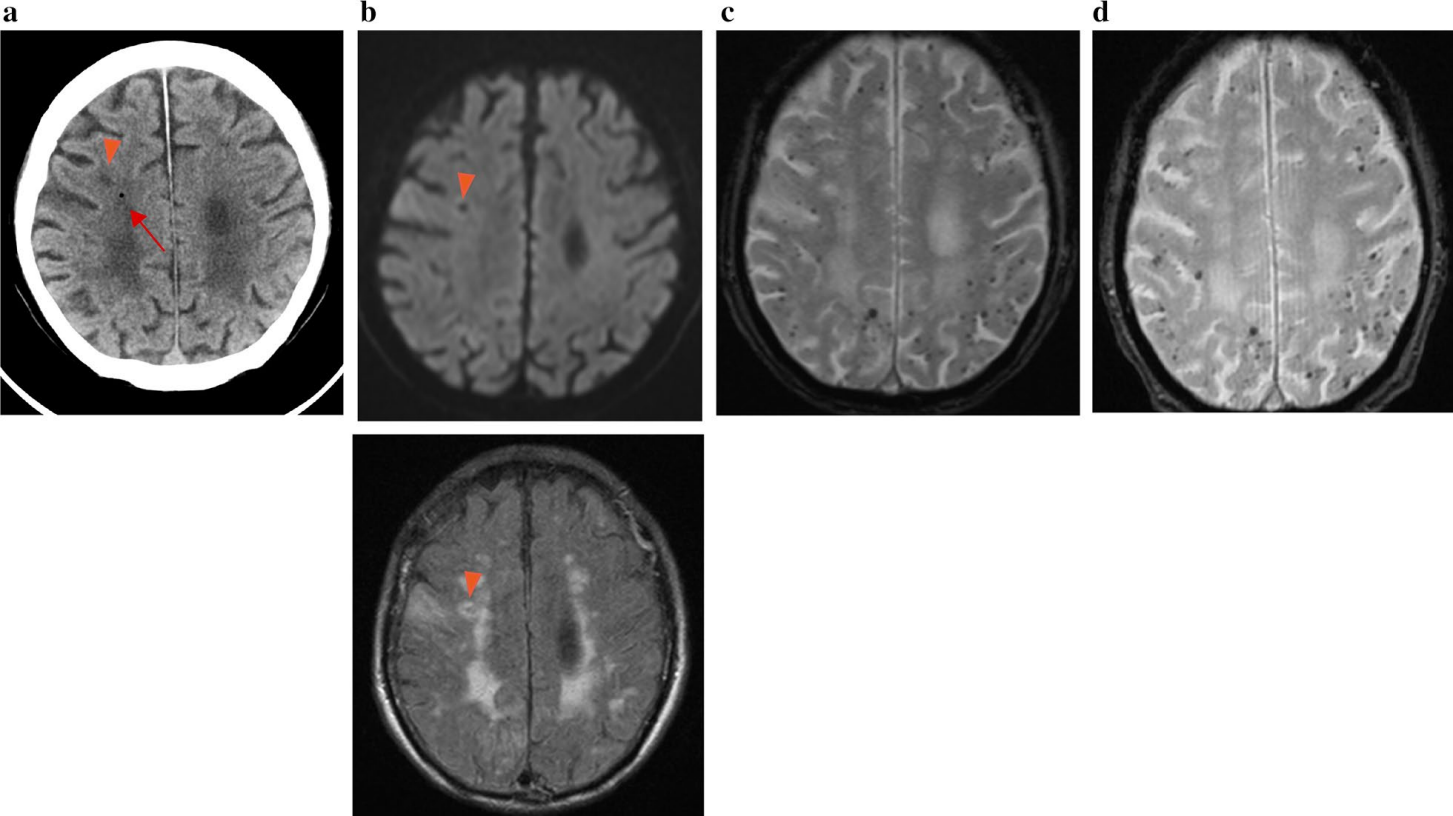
Brain CT and MRI of case 2. a Brain CT on admission shows a low-density spot in the right frontal lobe (red arrow) and old infarction (red arrowhead). b diffusion-weighted imaging (DWI) and fluid attenuated inversion recovery (FLAIR) images showed old infarction (red arrowhead), did not show obvious signals corresponding to the low-density spot on brain CT. c T2 star-weighted imaging (T2*WI) of MRI of case 2 on admission shows many hypointense signals in bilateral frontoparietal lobes. d T2 star-weighted imaging at 78 days after onset shows that the distribution of signals did not change compared with T2 star-weighted imaging on the admission day
